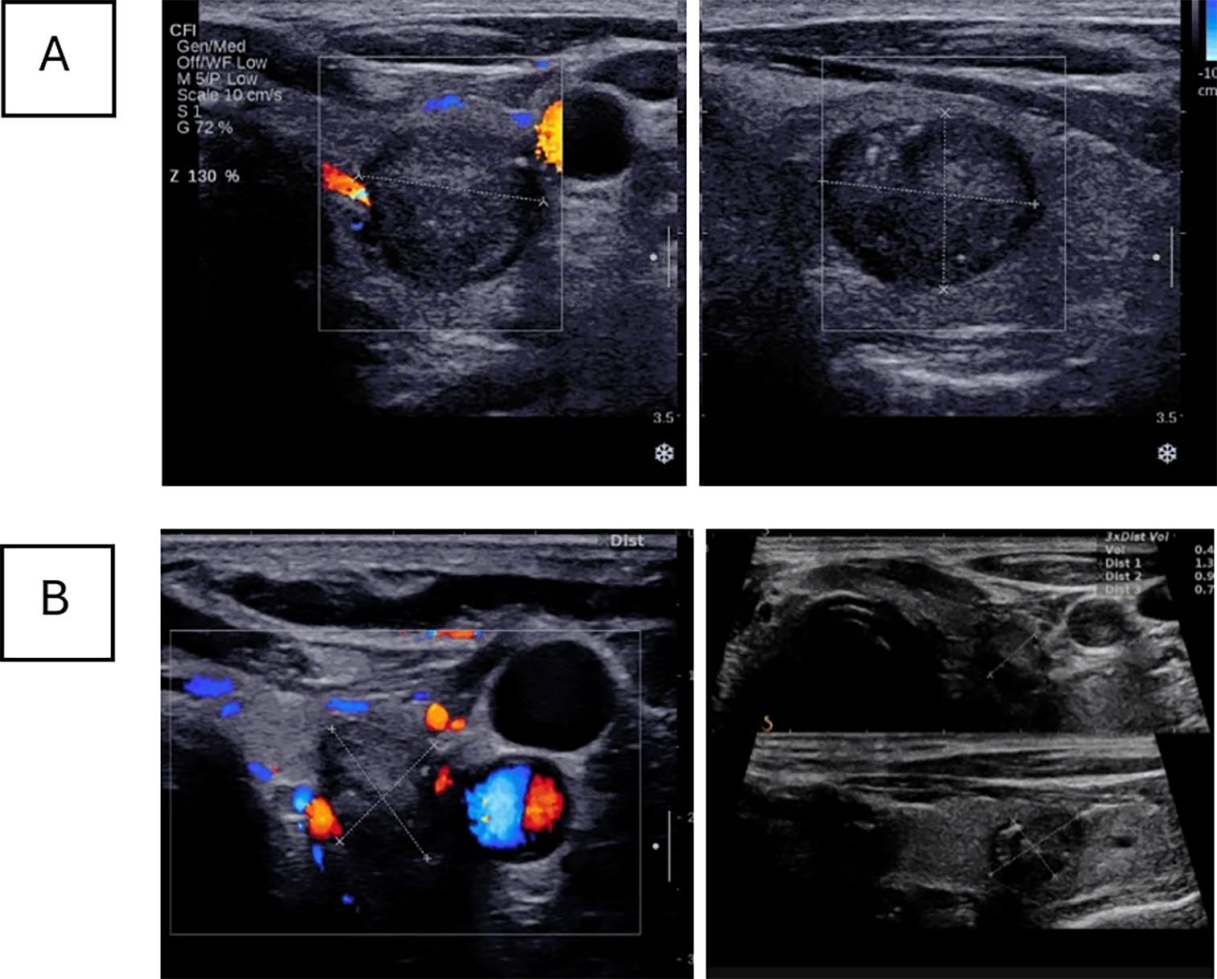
(A). Before the second ablation (6 months after the first RFA), there was a reduction in tumor size (13 × 10 × 10 mm). (B) Three months after the second ablation (9 months after the first RFA), the size of the thyroid nodule was 7.7 × 9 × 13.6 mm (0.49 cc) in thyroid ultrasonography.
